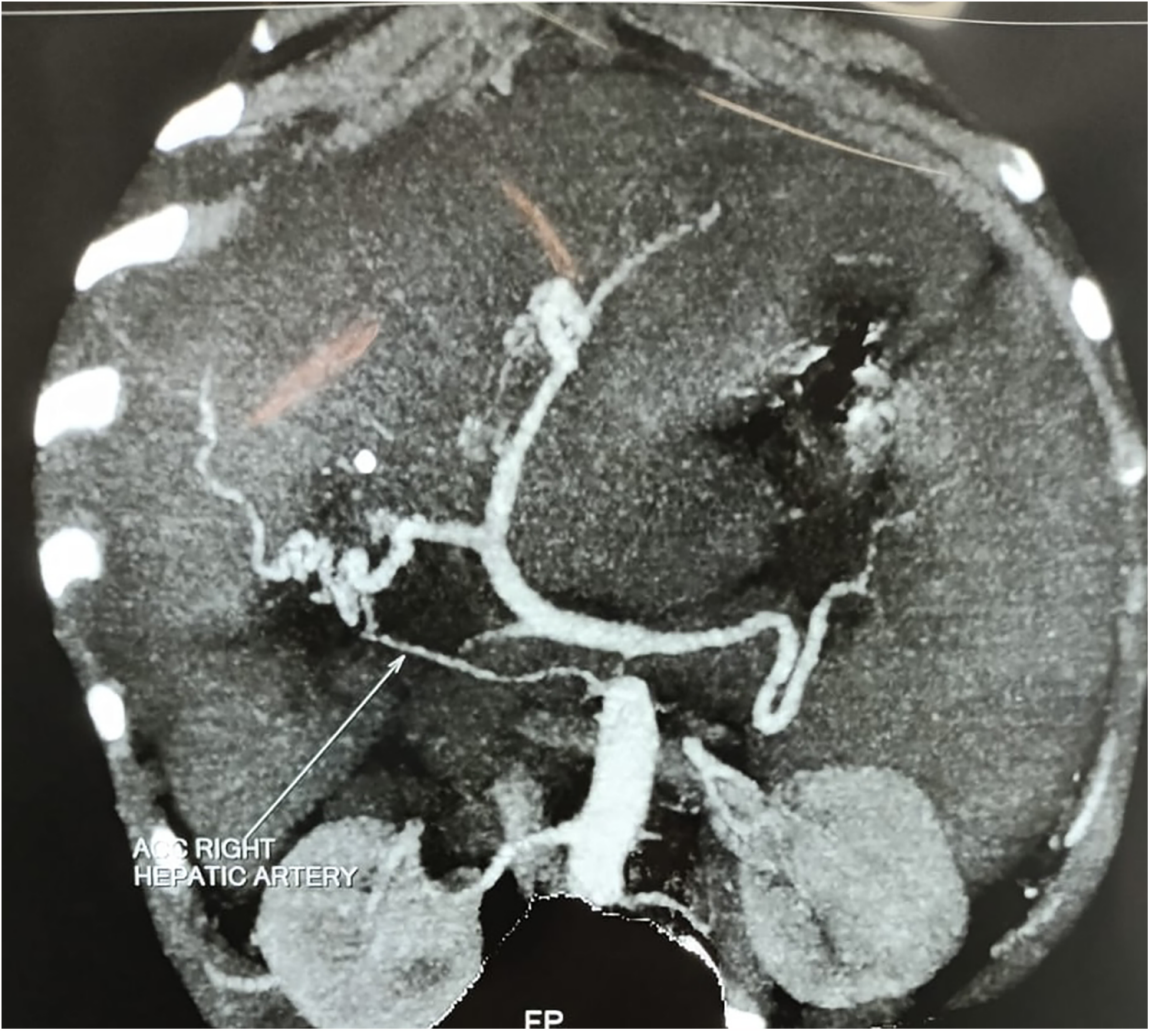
CT scan showing hepatomegaly and splenomegaly.
